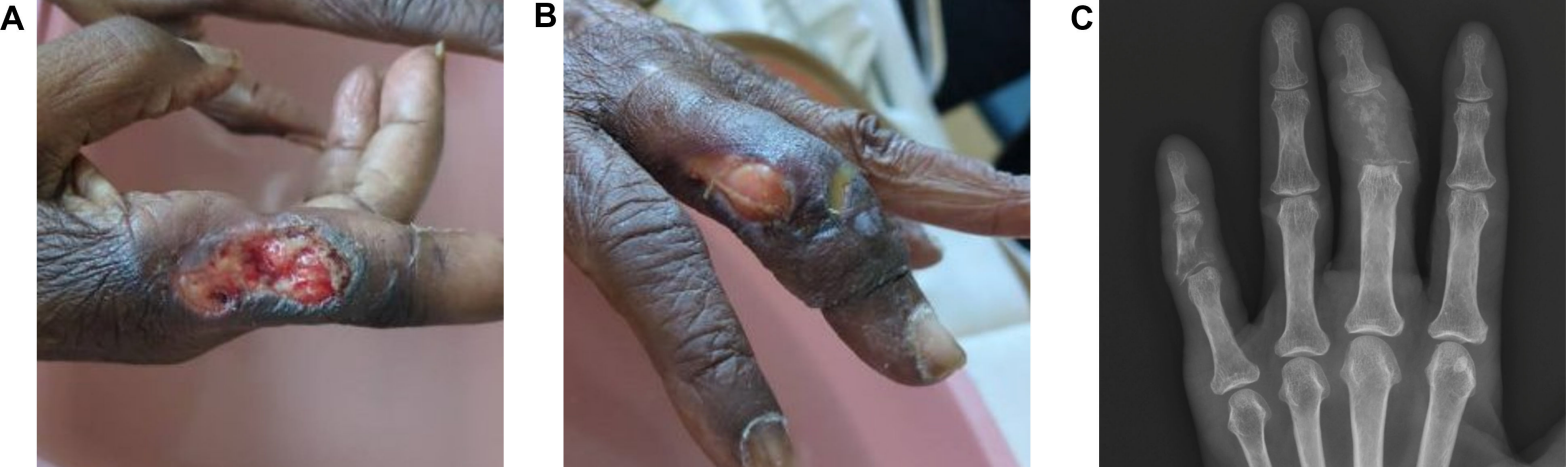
Clinical images. (A) Right hand ulcerative lesion on hospital admission. (B) Left hand ulcerative lesion on hospital admission. (C) Initial radiograph of the left hand showing osteomyelitis of the middle phalanx.
